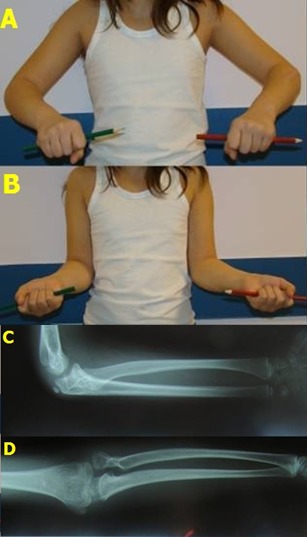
Clinical and radiologic check-up at two years after surgery. A. Maximum pronation; B. Maximum supination; C. Lateral X-ray – radial head in anatomic position; D. Anterior-posterior X-ray – normal relationship between the head of the radius and the humeral condyle.
